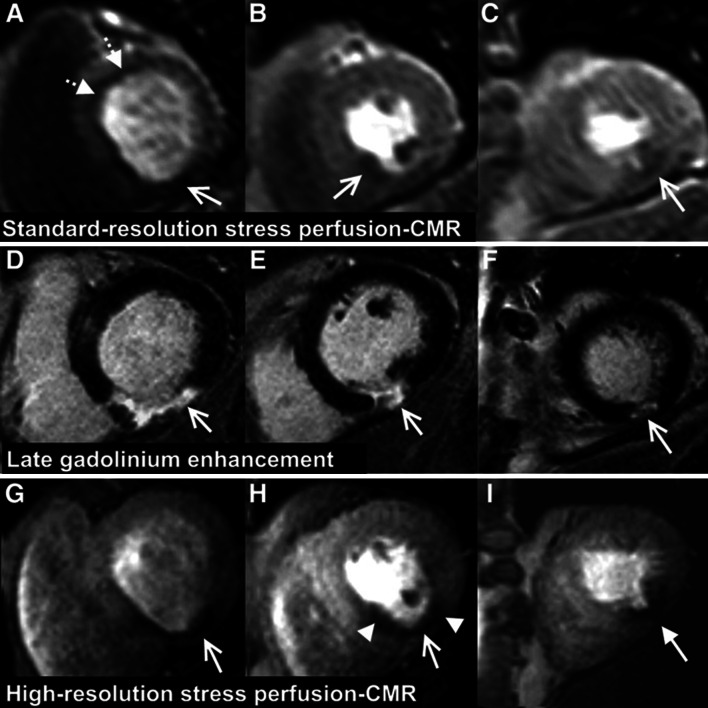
Examples of patients with ischemia acquired in typical late gadolinium enhancement, standard, and high-resolution perfusion MRI. *Arrows* indicate the inferior scar with thinning of the myocardium [30]
